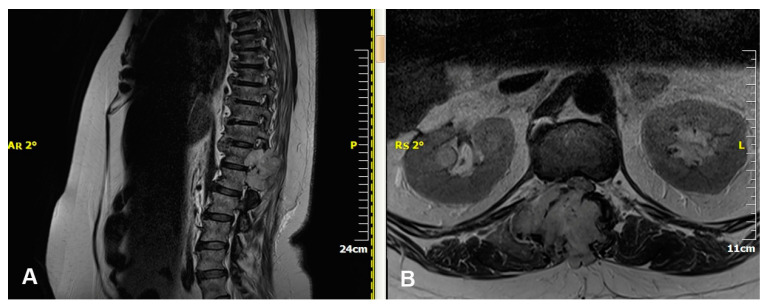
MRI of thoracolumbar renal metastasis. (A) Sagittal plane, (B) Axial plane.
